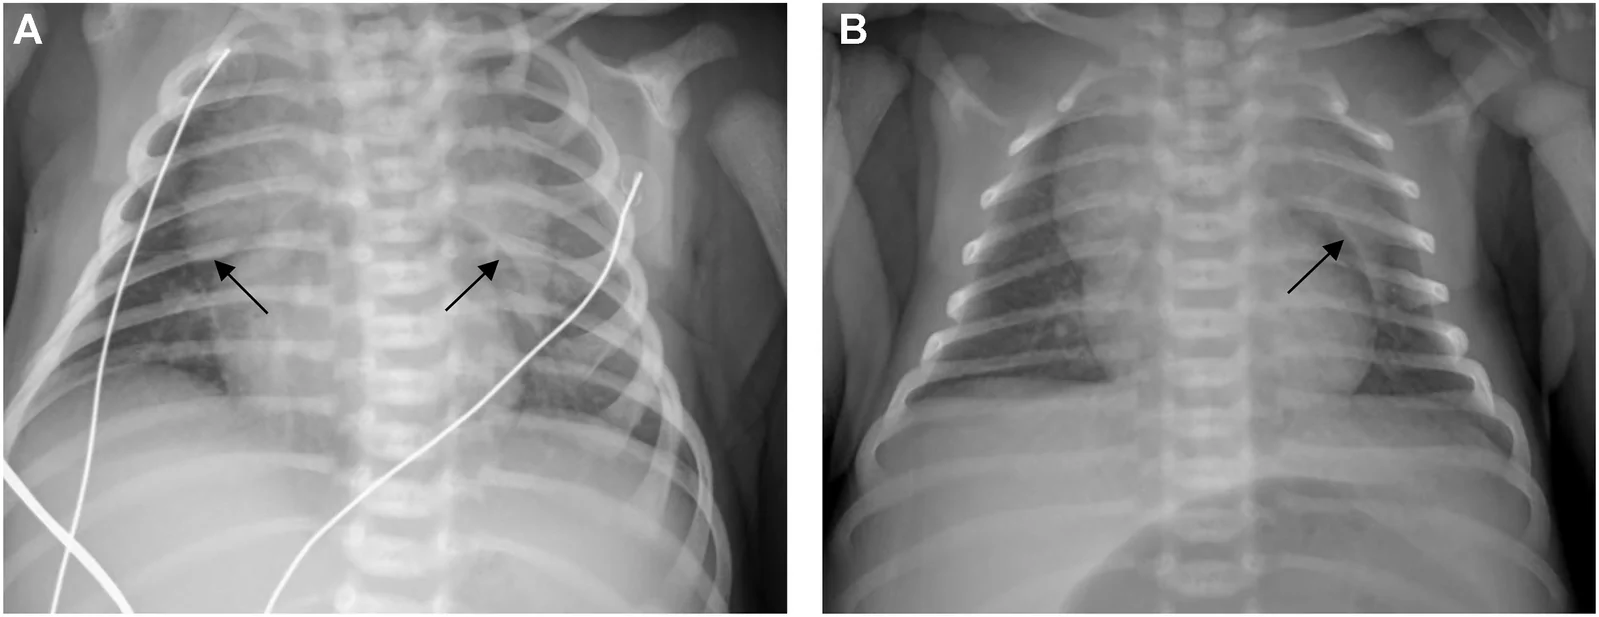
Frontal chest radiographs demonstrating neonatal pneumomediastinum. (A) Mediastinal air elevates the thymic lobes (arrows), producing the characteristic Spinnaker-sail sign. (B) Follow-up radiograph at day 5 of hospitalization showing persistent elevation of the left thymic lobe (arrow).
